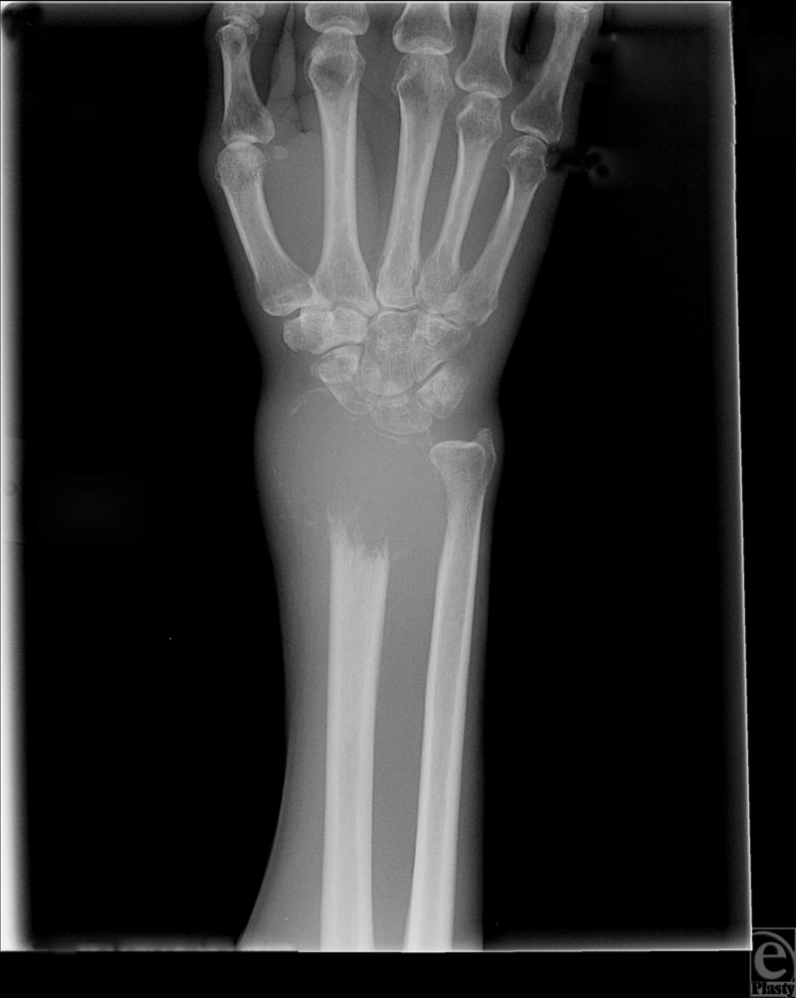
Plain radiograph of Giant Cell Tumor of the Wrist demonstrating an osteolytic lesion of the distal radius.
